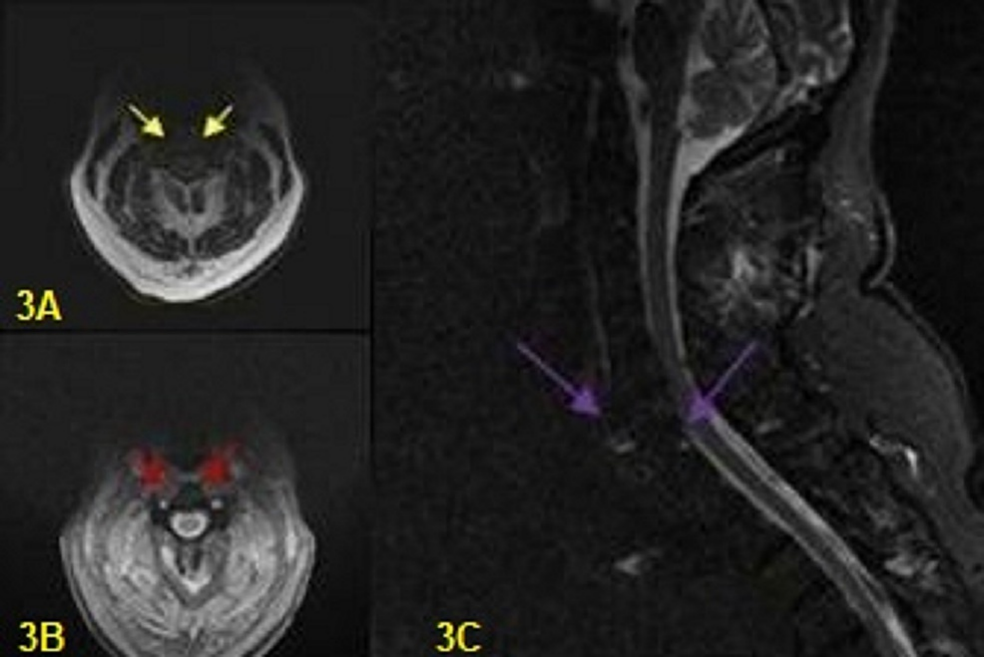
Magnetic resonance imaging (MRI) A 60-year-old man patient with cervical spine trauma who presented with severe pain of the cervical spine, headache, and numbness of his arms: (A) Magnetic resonance imaging (MRI) axial images (2D MERGE - Multiple Echo Recombined Gradient Echo) present linear enhancement signal intensity in C6 and C7 vertebras (yellow arrows); (B) Magnetic resonance imaging (MRI) axial images (3D COSMIC - Coherent Oscillatory State Acquisition for the Manipulation of Imaging Contrast) present linear enhancement signal intensity in C6 and C7 vertebras (red arrows); (C) Magnetic resonance imaging (MRI) sagittal STIR (Short Inversion Recovery) images present high signal intensity in the region of the suspicious fracture at the C6-C7 level (purple arrows)
